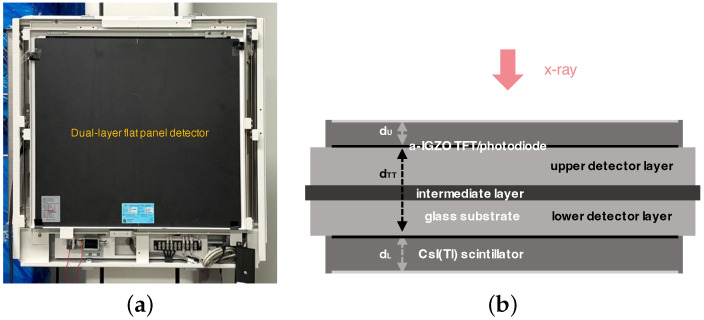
Structure of the dual-layer flat-panel detector (DFD) (DRTECH Co. Ltd., Seongnam, Republic of Korea, www.drtech.com (accessed on 27 July 2025)). The prototype DFD comprises stacked indirect-conversion detectors with CsI(Tl)-scintillator layers, where the pixel pitch is 0.14 mm/pixel and the image size is 3072 × 3072 pixels. (a) Appearance of DFD. (b) Structure of DFD. The glass substrates of the two detector layers are attached to each other. The lower detector layer is attached opposite to the direction of the incident x-ray [11]. The distance between a-IGZO TFT layers is $d_{\mathrm{TT}}$. The thicknesses of the upper and lower scintillator layers are $d_{U}$ and $d_{L}$, respectively. The pixels on the lower layer may not be in the same position as the pixels on the upper layer.
